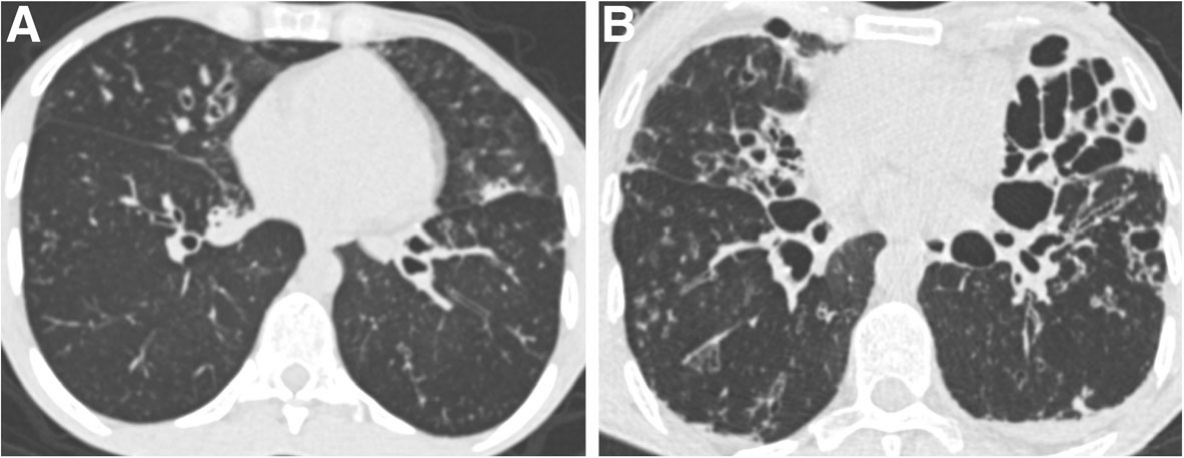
Different imaging features in patients with similar FEV_1_ values. a Patient with FEV_1_ = 38% predicted and moderate bronchiectasis predominantly affecting the middle lobe. The CT-density score based on MLD + 1SD was 7.79. b Patient with FEV_1_ = 38% predicted but much more severe bronchiectasis on visual assessment, especially in the lingula. The CT-density score based on MLD + 1SD was 12.75. These two examples show that CT imaging provides additional information to spirometry, especially regarding regional disease distribution (homogeneous versus heterogeneous), and the severity of bronchiectasis, which correspond to irreversible changes
